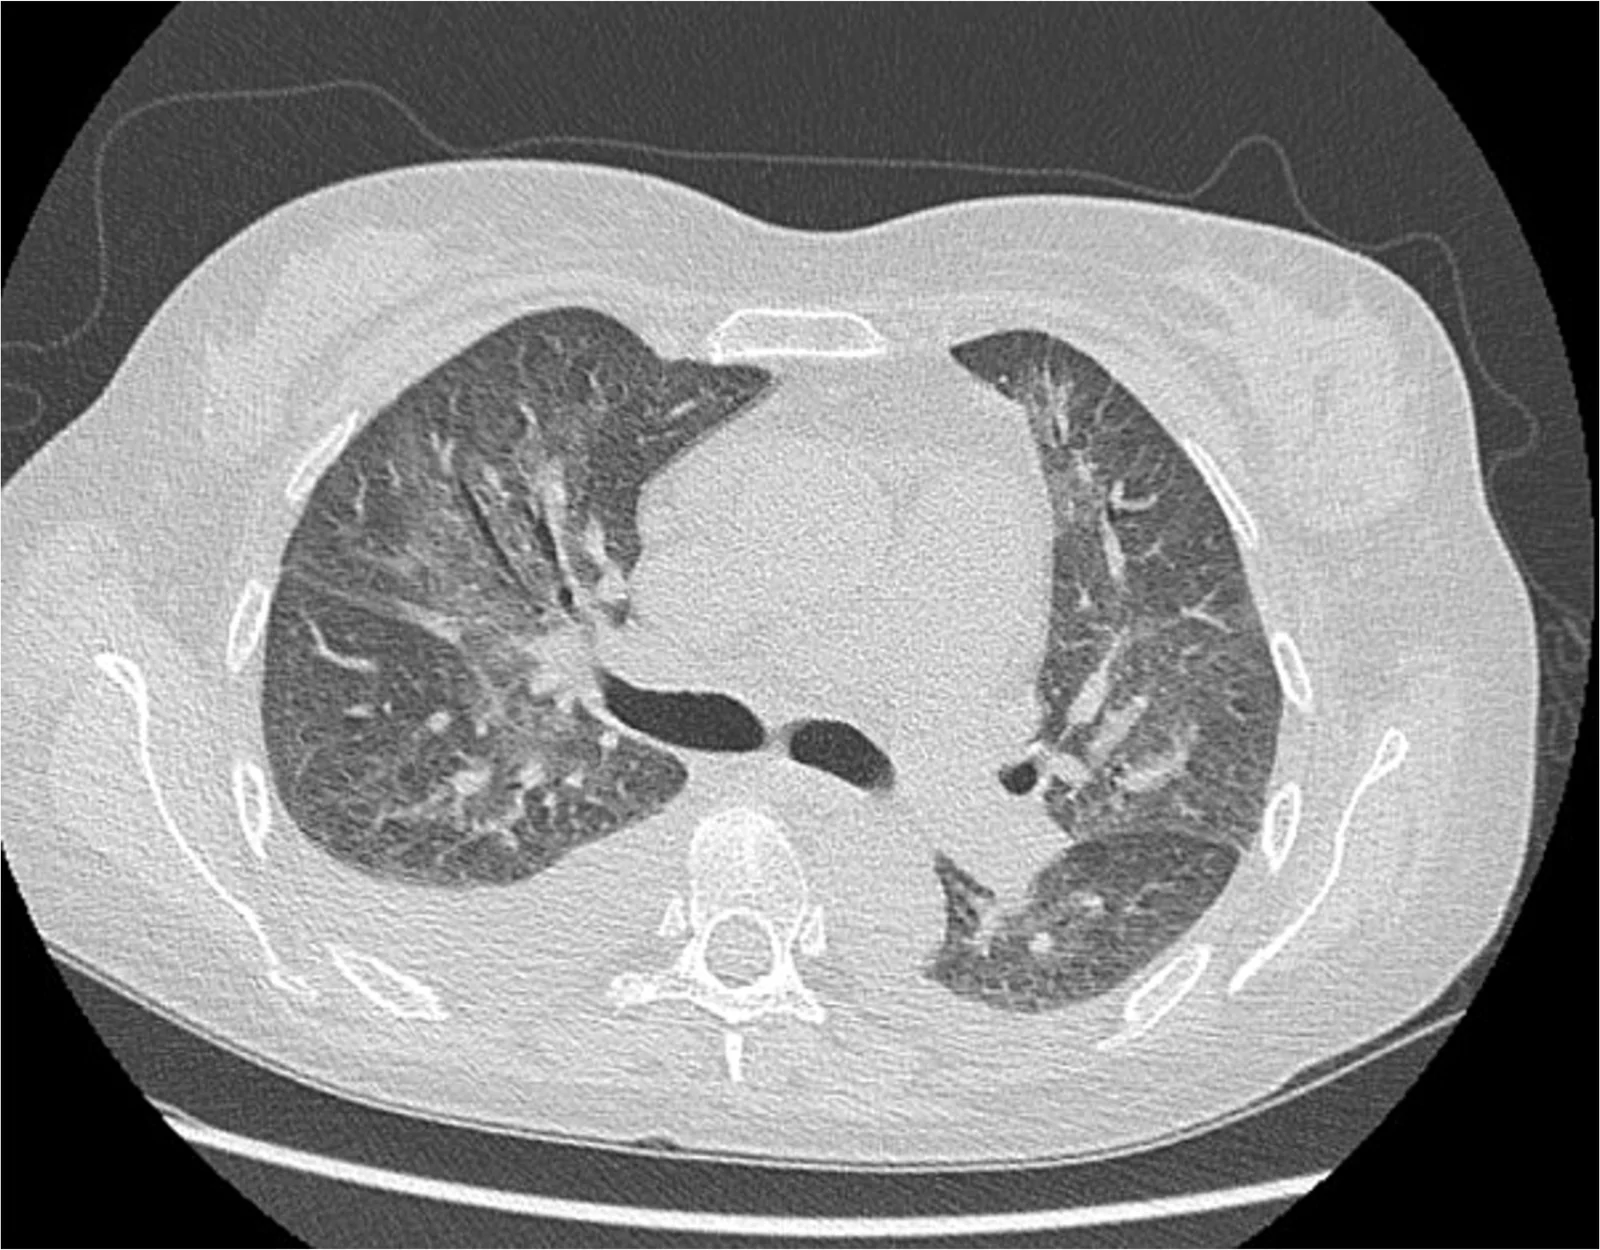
High resolution CT chest showing bilateral consolidation, atelectasis, ground-glass changes and bilateral pleural effusions.
